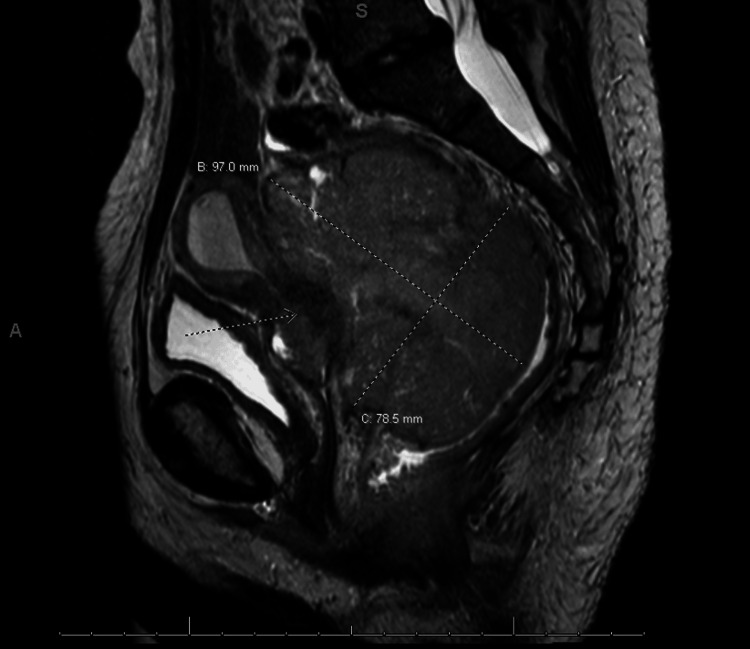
Sagittal MRI at diagnosis reveals a large pelvic mass measuring 9.7 cm by 7.8 cm, compressing the uterus, rectum, and bladder The arrow is pointing to what remains of the normal cervix.
